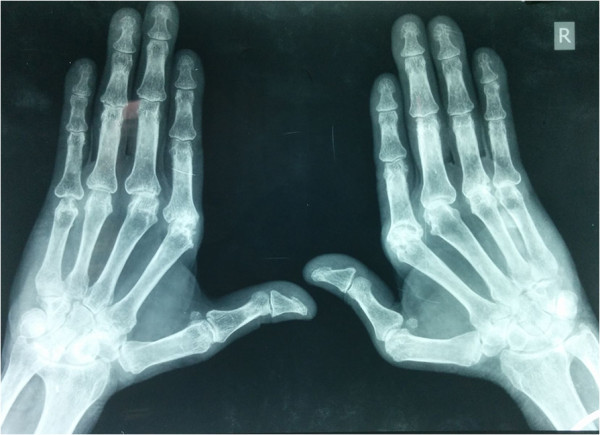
Radiograph of both hands. Note the prominent erosion in the left 2nd MCP.
